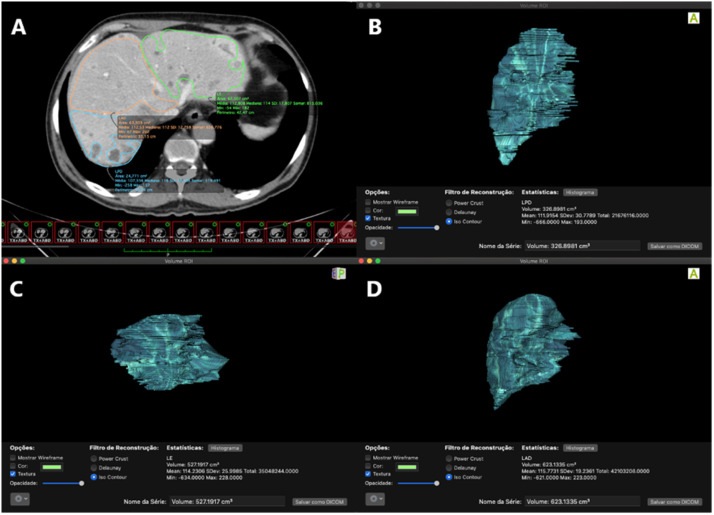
(A) Pre-drainage CT axial section, with selected areas for the left lobe and anterior and posterior segments of the right lobe (note that areas with metastatic lesions were excluded); (B) Volumetric calculation of the posterior segment of the right lobe; (C) Left lobe volumetric calculation; (D) Volumetric calculation of the anterior segment of the right lobe.
